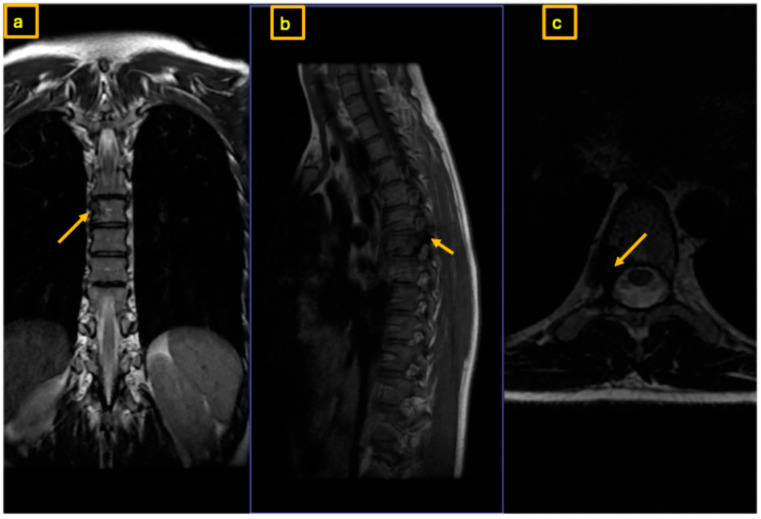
Postoperative MRI of the thoracic spine at 3-month follow-up. The yellow arrow indicates recurrence of the nidus at the root of the right T7 pedicle, with possible involvement of the vertebral body: (a) coronal view, (b) sagittal view, and (c) axial view.
